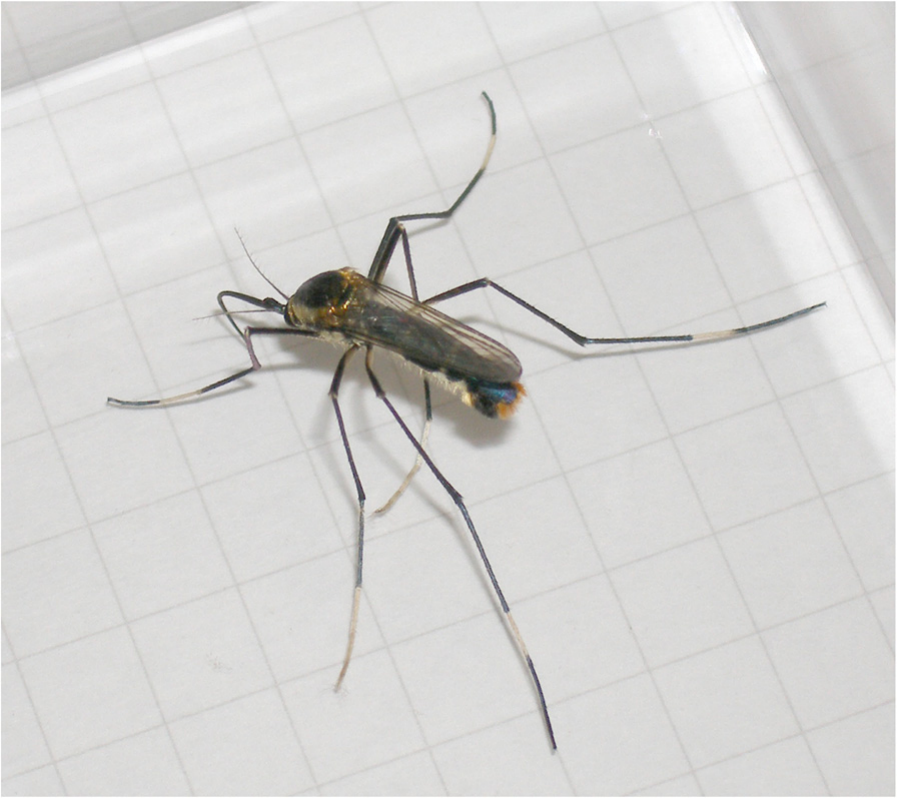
Picture of the giant mosquito (*Toxorhynchites towadensis*) that was used for the X-ray experiment. The size of the mesh is 5 mm
